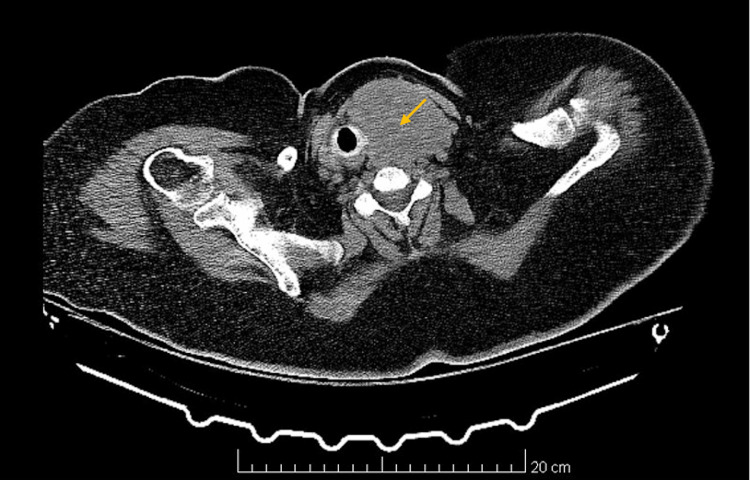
Chest computed tomography: heterogeneous mass in the left cervical region.
